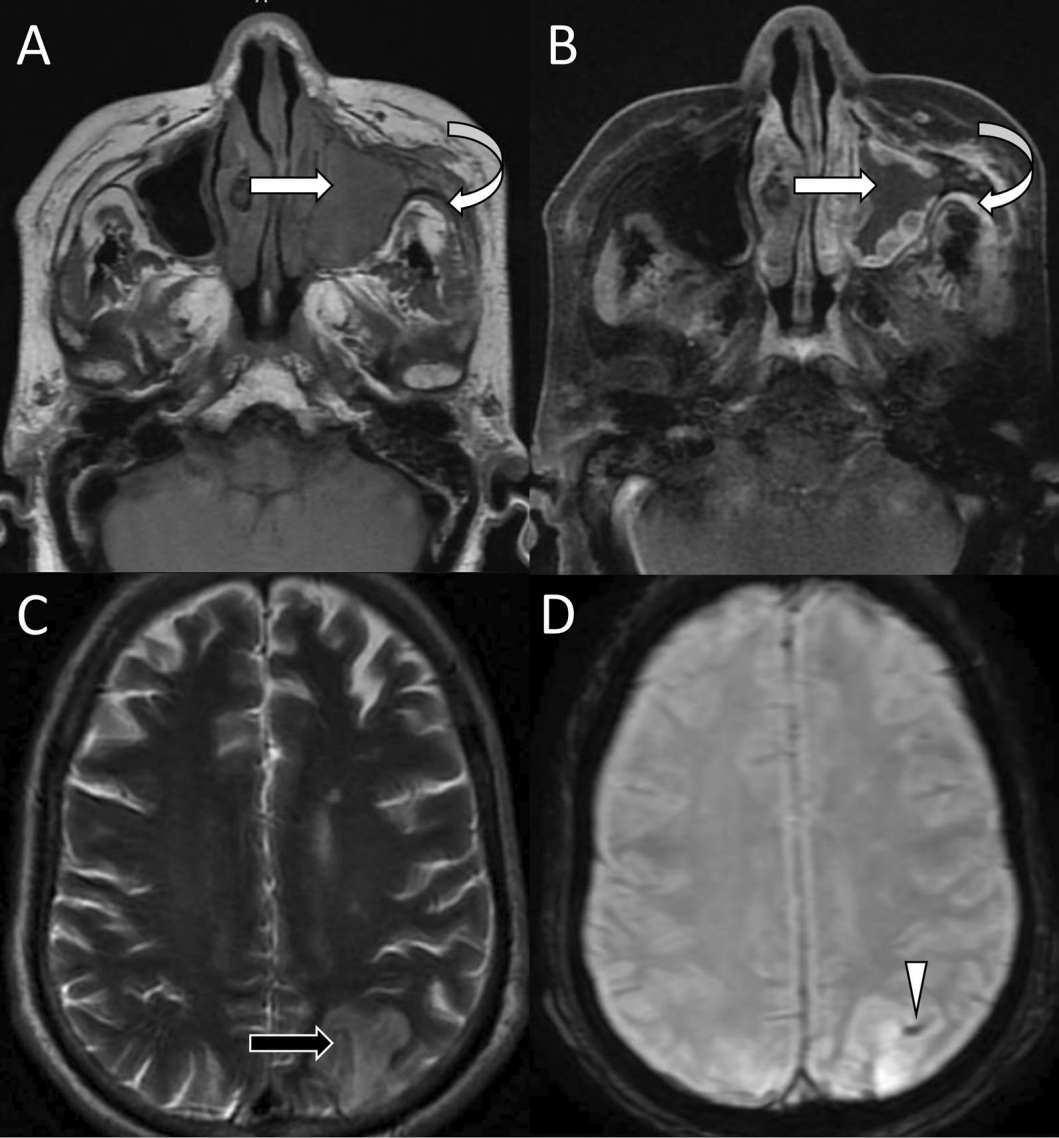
Rhinocerebral mucormycosis with late cerebritis. Axial T1-weighted image (A) shows soft tissue in the left maxillary sinus (straight white arrow) with extension into left retroantral fat (curved white arrow). Contrast-enhanced axial T1-weighted fat-saturated image (B) shows peripheral enhancement of the maxillary lesion with intense enhancement of retroantral fat. Axial T2-weighted (C) and susceptibility-weighted (D) images show a heterogeneous hyperintense lesion in the left parietal lobe (black arrow) which shows peripheral foci of blooming (arrowhead)
